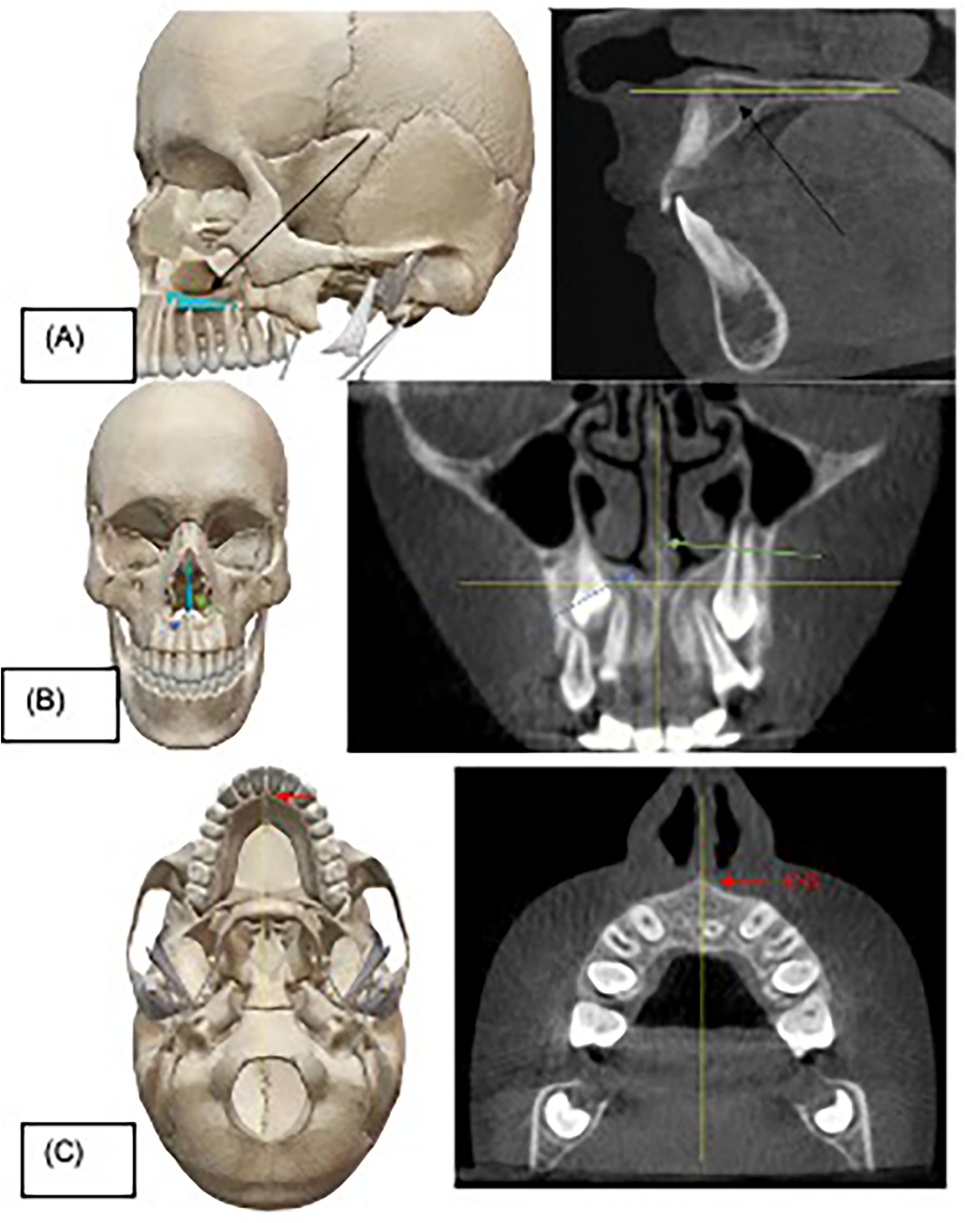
Head position adjustment before taking measurements. (A) Dry skull showing the palatine process of maxilla (black arrow) that was used as a reference in sagittal view of cone-beam computed tomography (CBCT) image. (B) Dry skull showing the nasal septum (green arrow) and nasal floor (blue arrow) which were used as references in coronal view of CBCT image. (C) Dry skull showing the anterior nasal spine (ANS) (red arrow) used to help adjust the midsagittal plane to be perpendicular to the ground. Yellow lines reflect the correct head position in all three planes.
